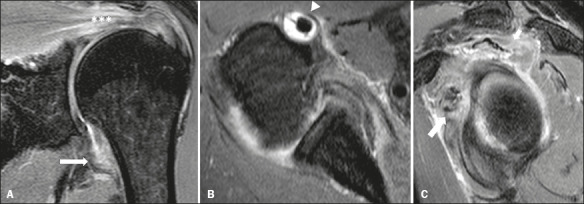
MRI of the left shoulder in a 70-year-old male with PMR. A: Coronal
